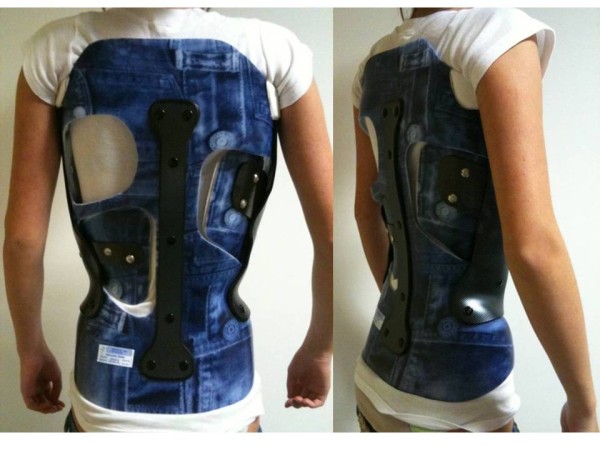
Face and profile x-rays without brace. Double-major scoliosis.
